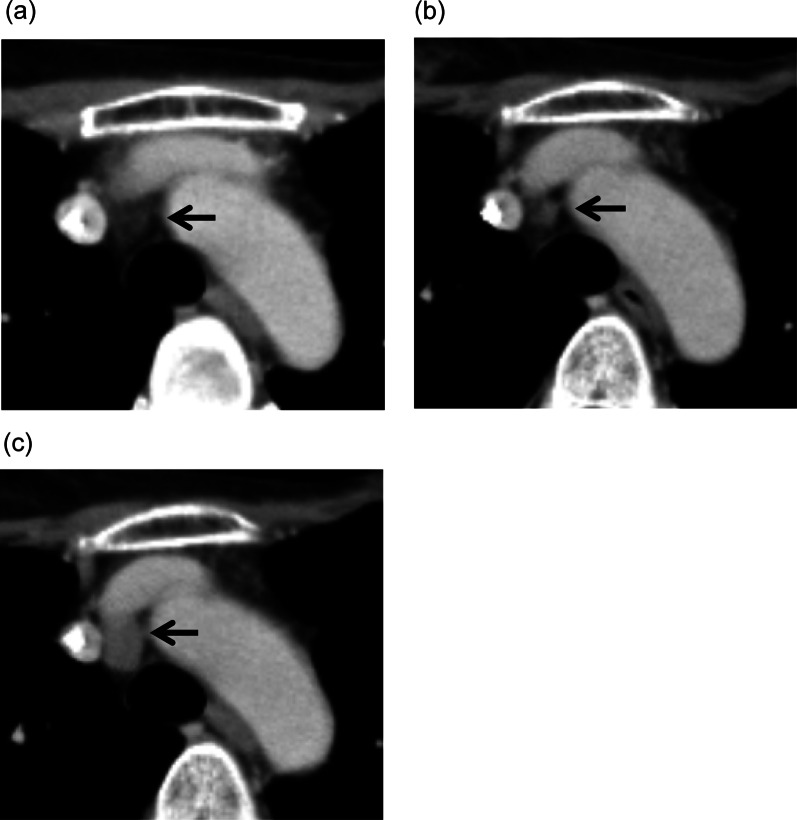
Chest CT scans obtained in the a first year, b second year, and c third year after surgery. The scans showed that the mediastinal nodule had been gradually growing for 3 years
